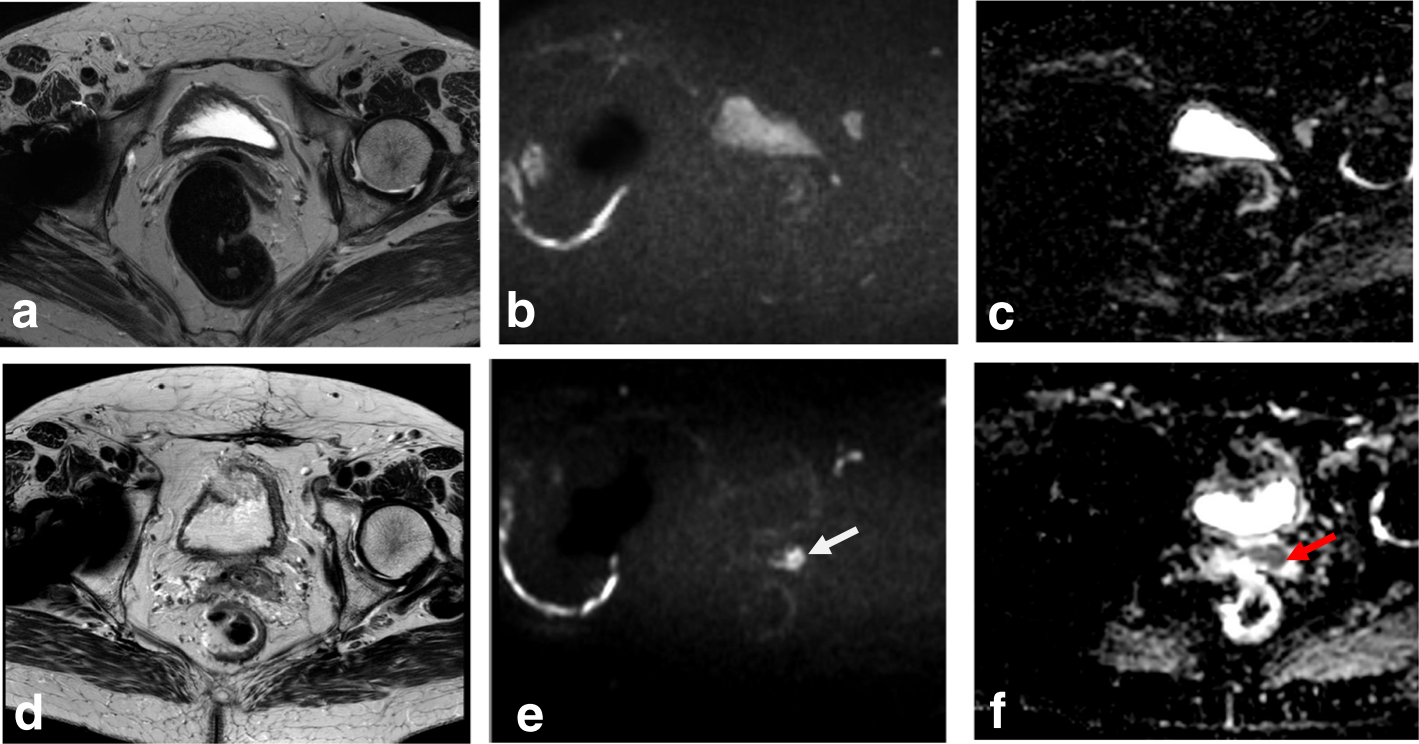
MRI technique artifacts. a Axial oblique T2WI. b, c DWI (b: 1000 and ADC map). Right hip prostheses and rectal air prevent detection of a small cervical tumor. d–f Axial oblique T2WI and DWI. After rectal air removal and change of phase direction, a tiny cervical cancer tumor is visible (arrows)
